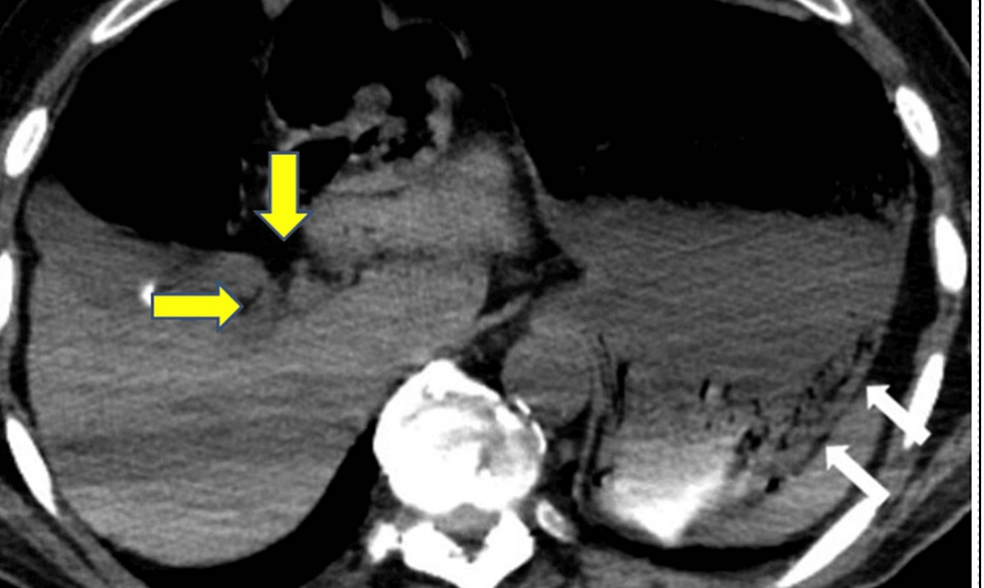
Axial view of CT abdomen and pelvis without contrast White arrows: Distended stomach with questionable pneumatosis within the wall of the fundus; Yellow arrows: Portal venous gas within the liver
